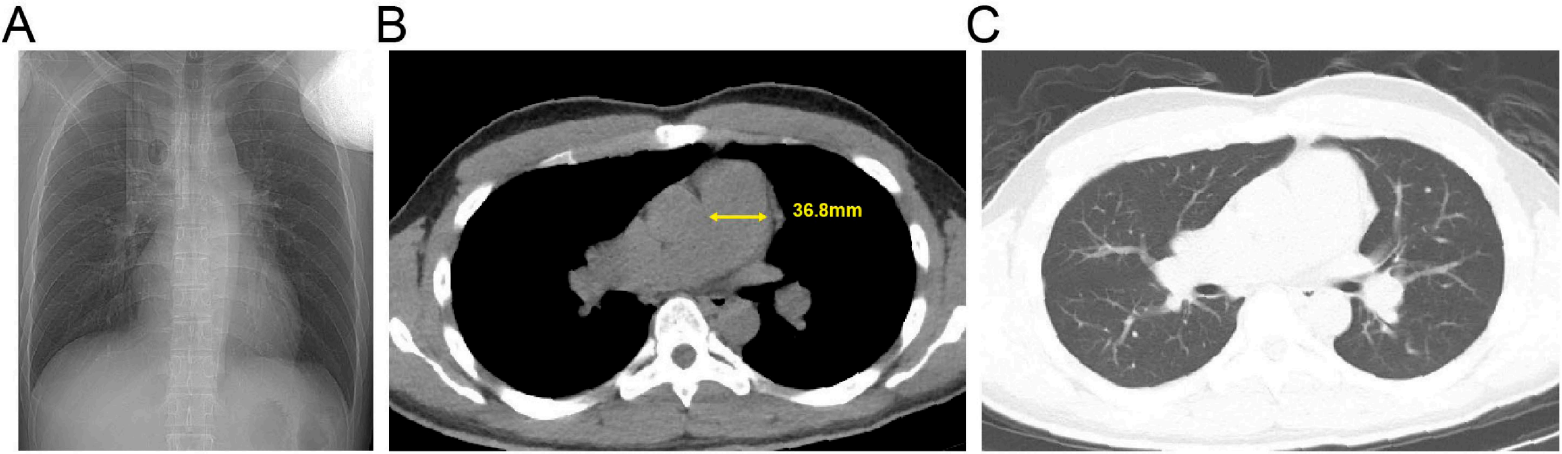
Admission chest computed tomography (CT) of the patient with pulmonary hypertension. (A) Axial non-contrast image. (B) Mediastinal window view; the yellow solid line indicates the transverse diameter of the main pulmonary artery. (C) Lung window view.
